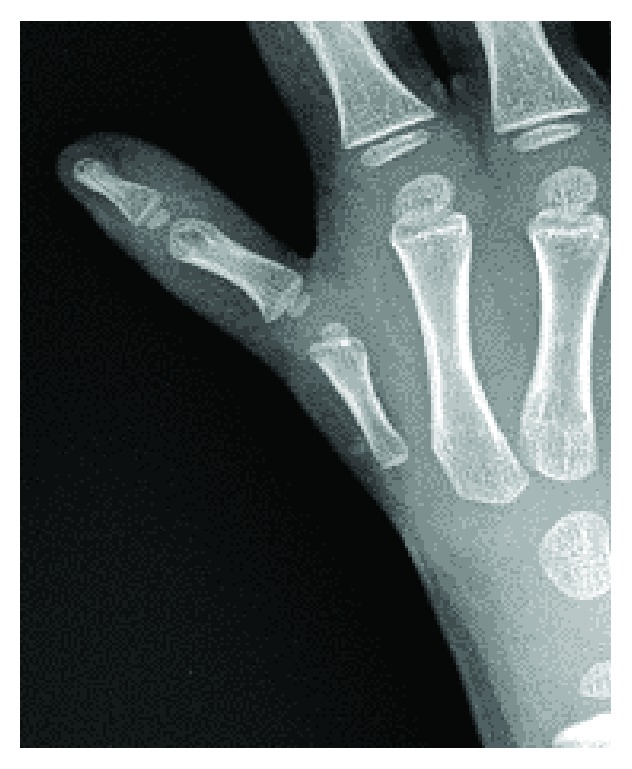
Radiographs demonstrating the type IIIB hypoplastic thumb.
